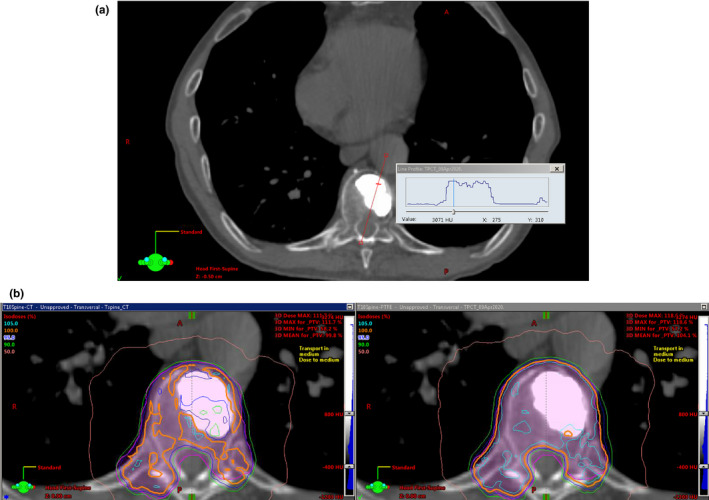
Clinical case involving SBRT to the T10 vertebral body, displayed using a bone window. (a) CT HU profile, and (b) dose distributions showing differences of 5 to 10% in target dose using cement density based on CT HU (left) and PTFE (right).
